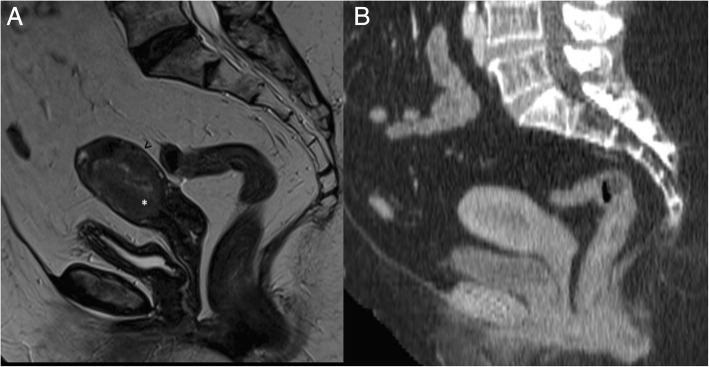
A 66-year-old woman with endometrioid adenocarcinoma, grade 3. Sagittal T2-weighted image shows an intermediate-signal intensity endometrial tumour invading > 50% of the myometrium (a), with disruption of the low T2 signal junctional zone (white asterisk) and preservation of the low signal band of the outer myometrium (black arrowhead). Sagittal post contrast CT (b) in the same patient, performed for evaluation of distant metastases, does not discriminate the extent of myometrial infiltration
